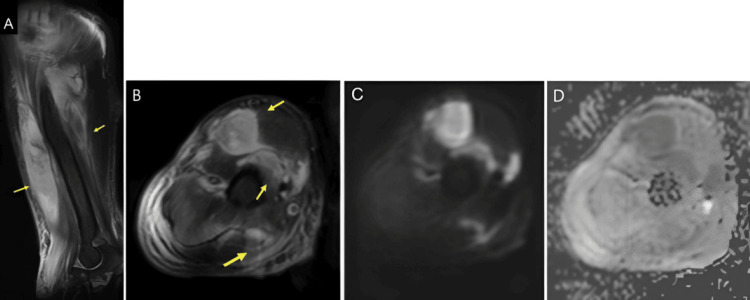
MRI of the right upper limb On day 8 after admission, intramuscular lesions with high signal intensity in the right branchial biceps muscle and brachial triceps muscle are depicted on sagittal and axial fat suppression T2WI MRI (A, B) and DWI (C), and ADC values are relatively low (0.95-1.403) (D), indicative of abscess formation. ADC, apparent diffusion coefficient; DWI, diffusion-weighted imaging; T2WI, T2-weighted imaging
